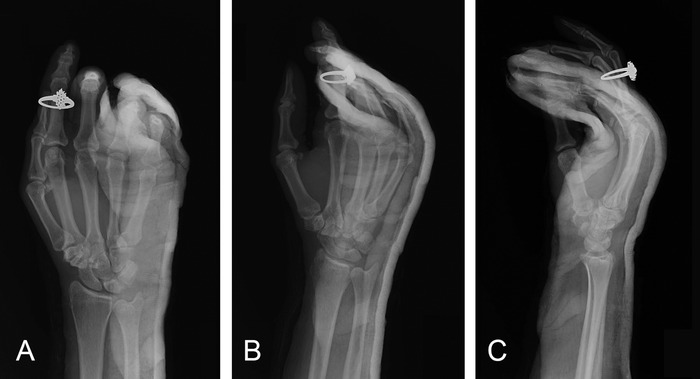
Postreduction right hand posteroanterior (A), oblique (B), and lateral (C) x-rays show adequate alignment of the fifth carpometacarpal joint.
